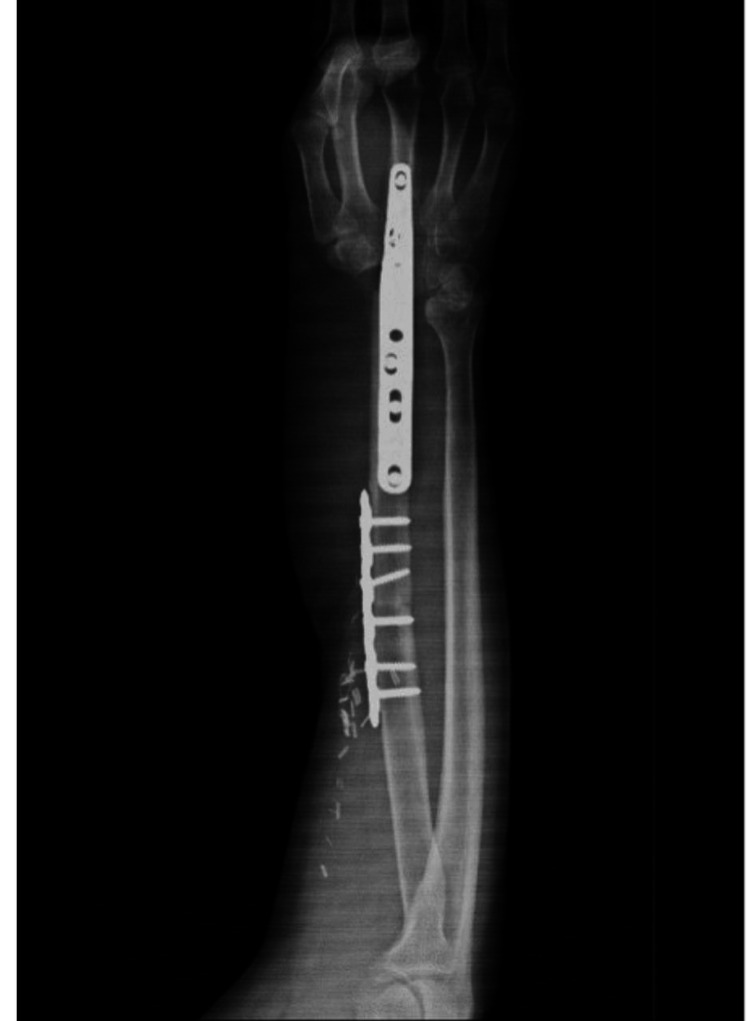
Radiograph at month three showing progressive flap integration with adequate consolidation and maintained stability of the fixation system
